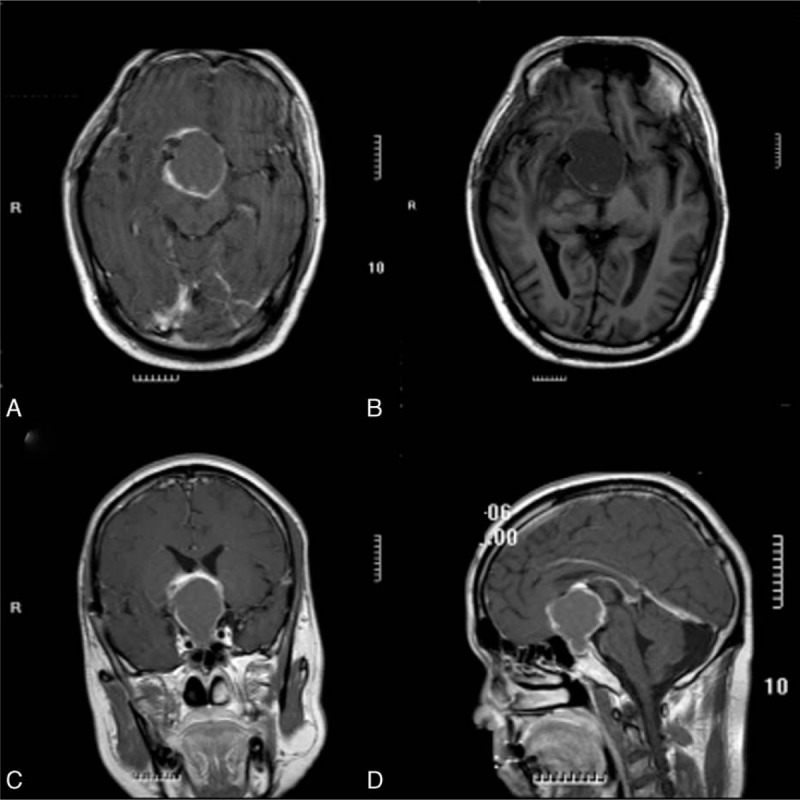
Magnetic resonance imaging showed cystosolid lesion with distinct border. The lesion was in size of 3.5 cm × 3.5 cm × 4.3 cm, with treatment of herbal decoction alone (August 2013) (A–D).
